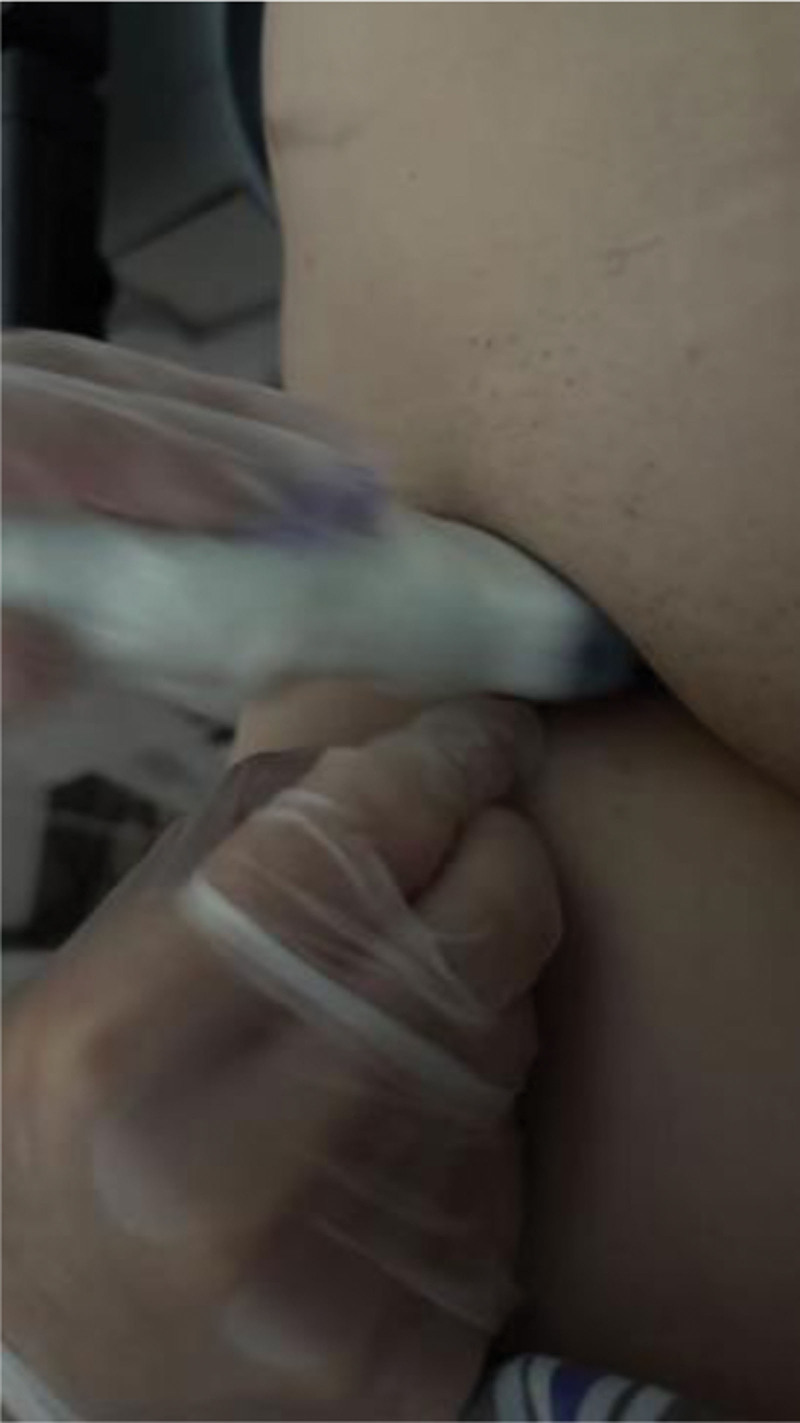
Preoperative ultrasound localization.
